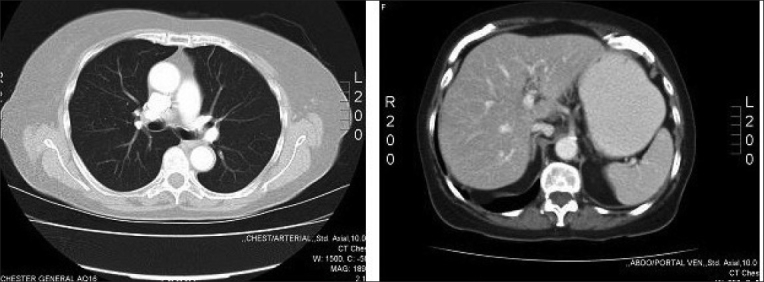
CT scan of chest and abdomen: Normal with no signs of metastatic lesions
